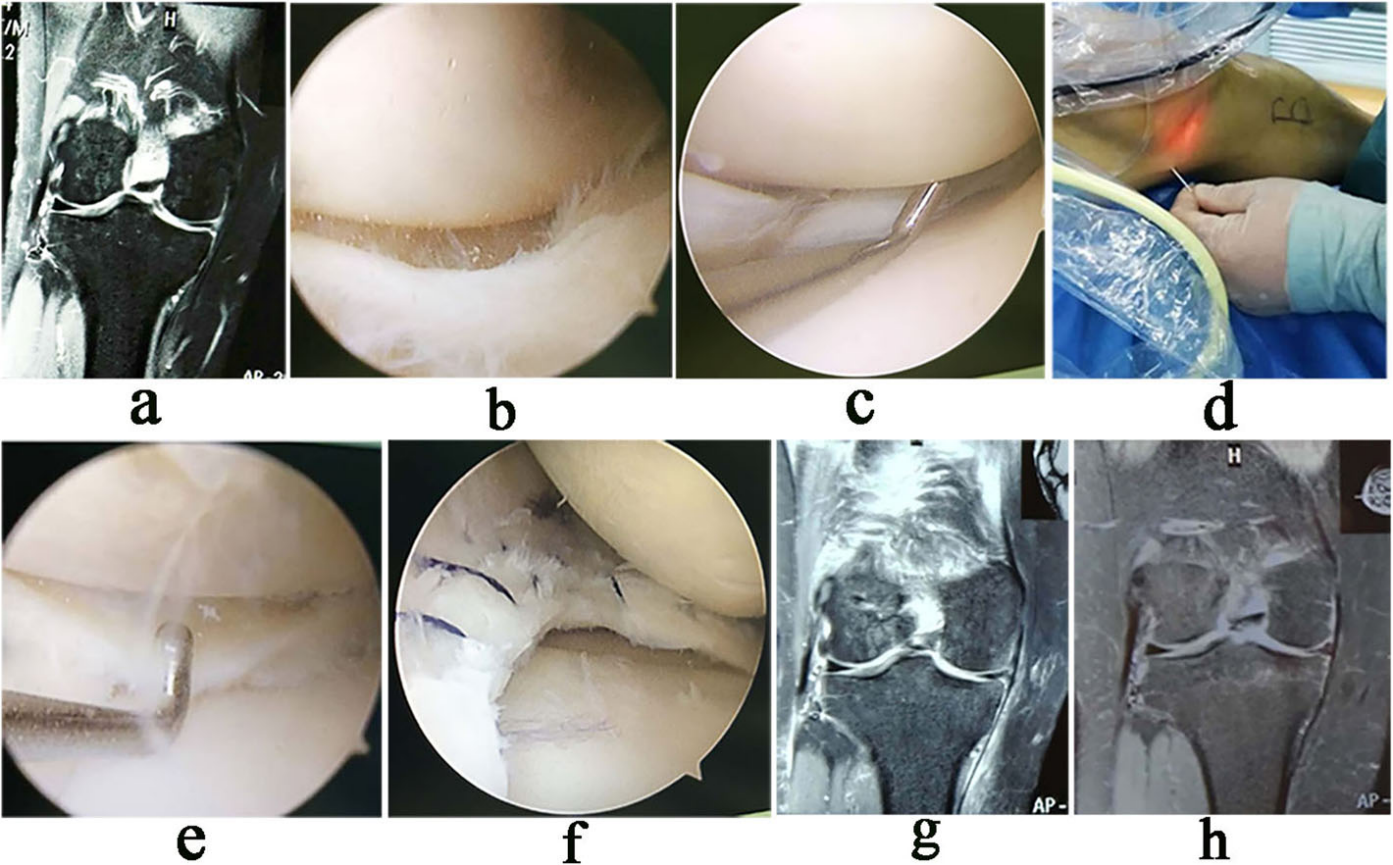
Arthroscopic pie-crusting release of PMC for meniscus suture. a Preoperative MRI showed a bucket-handle tear of the medial meniscus. b Displacement of the injured meniscus under arthroscopy. c The injured meniscus was reduced, but the probe tip could not pass vertically through the medial gap. d Multi-point release of the PMC. e After release, the probe tip could pass vertically through the narrow gap. f The meniscus was sutured, with no iatrogenic cartilage injury. g Postoperative MRI showed a normal meniscus shape and mild iatrogenic injury of MCL. h After 18 months follow-up, MRI showed healing of the meniscus and MCL
